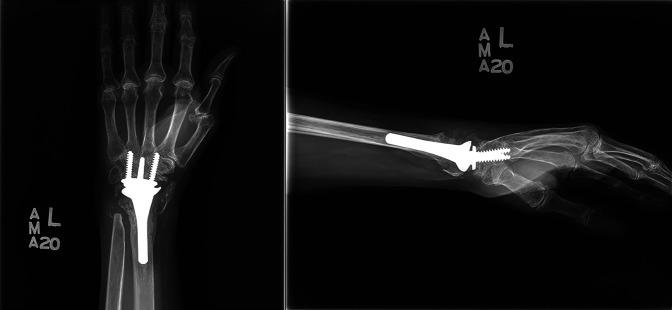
Postoperative AP and lateral radiographs of the left wrist after revision total wrist arthroplasty.
